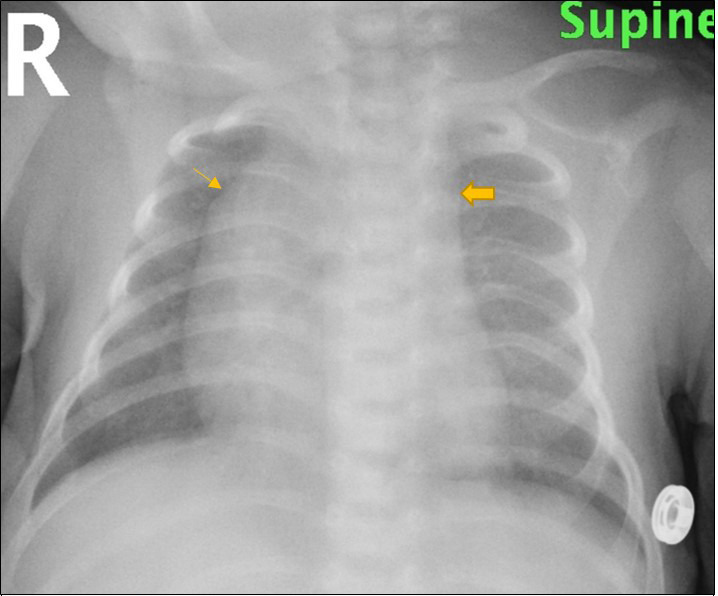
Chest X-ray frontal view shows straightening of the left cardiac border with loss of left aortic contour (block arrow) and prominent right para tracheal soft tissue (single arrow)
